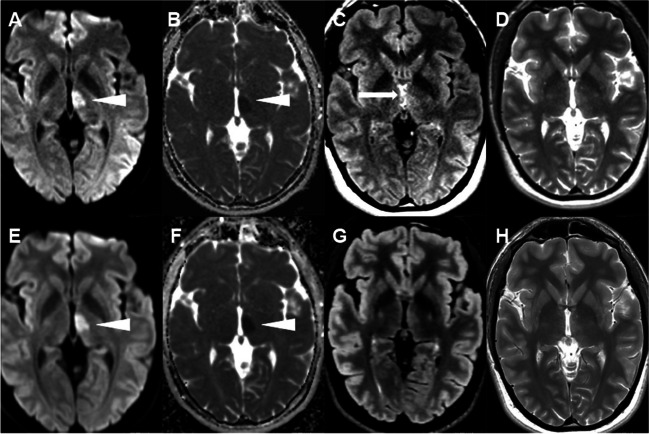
Images in a 27-year-old man who presented with right-sided weakness and dysphasia (NIHSS 6) at wake-up. CT without arterial occlusion. MRI was performed to detect DWI/T2-FLAIR mismatch. Axial DWI, ADC, T2-FLAIR, and T2-weighted images, A–D NeuroMix upper row and E–H cMRI lower row. A, E A high-signal DWI lesion with a corresponding low signal on B, F ADC is visible in the left thalamus with similar conspicuity on NeuroMix compared to cMRI (arrowhead). C, D, G, H On T2-FLAIR and T2-weighted images, a subtle high signal on NeuroMix and cMRI is present in the DWI lesion area with low conspicuity on both methods. T2-FLAIR abnormality is slightly more visible on cMRI due to a higher signal-to-noise ratio on cMRI and C adjacent high-signal cerebrospinal fluid pulsation artifacts in the third ventricle that are present only on NeuroMix T2-FLAIR (arrow). The clinical radiological interpretation was acute infarction with DWI/T2-FLAIR mismatch, and thrombolytic treatment with intravenous alteplase was given after MRI with a reduction of symptoms (NIHSS from 6 to 2)
